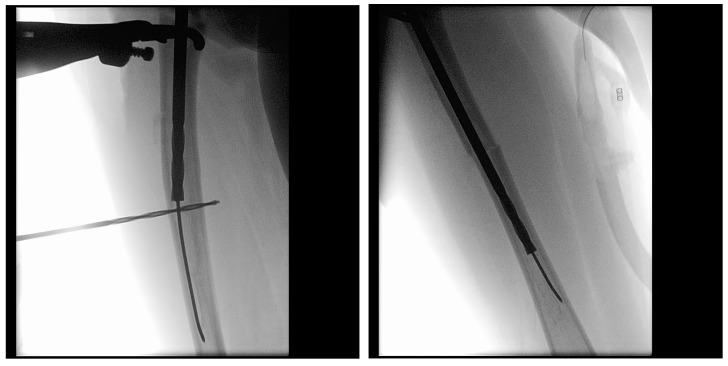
Intraoperative X-rays showing the second CORA and realignment with the continuation of the reaming.
